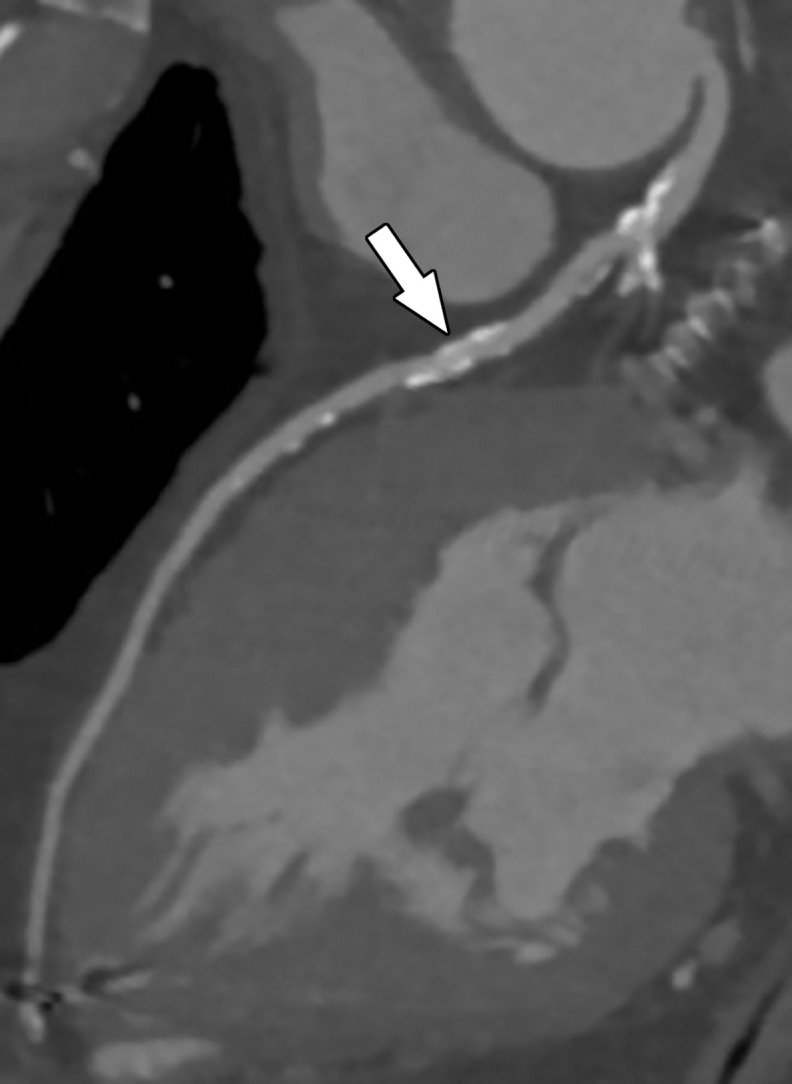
Referral for coronary CT as part of a preoperative evaluation in a 60-year-old man with a history of aortic valve replacement and complete heart block after dual-chamber pacemaker placement. (a) Curved multiplanar reformatted CCTA image of the LAD coronary artery shows a moderate (50%–69%) coronary stenosis (arrow) within the proximal LAD. (b) FFR CT image shows an FFR value of 0.72 distal to the moderate stenosis in the proximal LAD, compatible with a functionally significant coronary stenosis.
